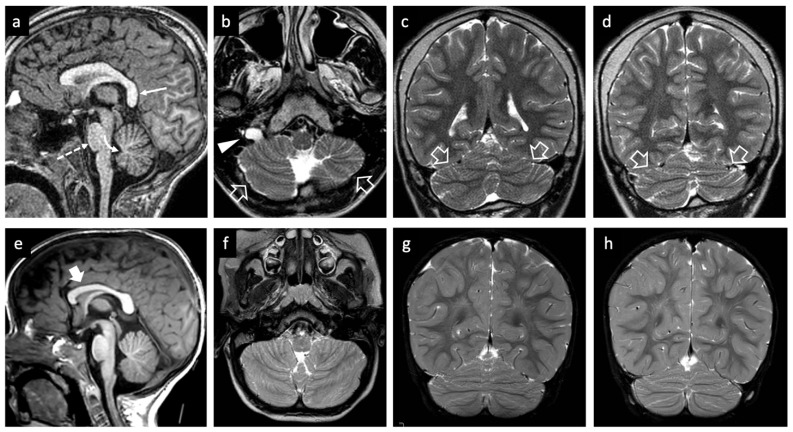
Brain MRI studies of proband 1 at 12 years of age (a–d) and of proband 2 at 1 year and 9 months (e–h). (a) The sagittal T1-weighted image reveals a vertical splenium (arrow) associated with a small pons (dashed arrow) and hypoplastic vermis with low-lying fastigium (curved arrow). (b–d) Axial (b) and coronal (c,d) T2-weighted images demonstrate a smaller left cerebellar hemisphere with bilateral abnormal orientation and depth of the cerebellar sulci (empty arrows). Note the marked dilatation of the right hypoglossal canal (arrowhead). (e) The sagittal T1-weighted image shows hypoplasia of the anterior portions of the corpus callosum (thick arrow). (f–h) Axial (f) and coronal (g,h) T2-weighted images reveal a normal cerebellum.
